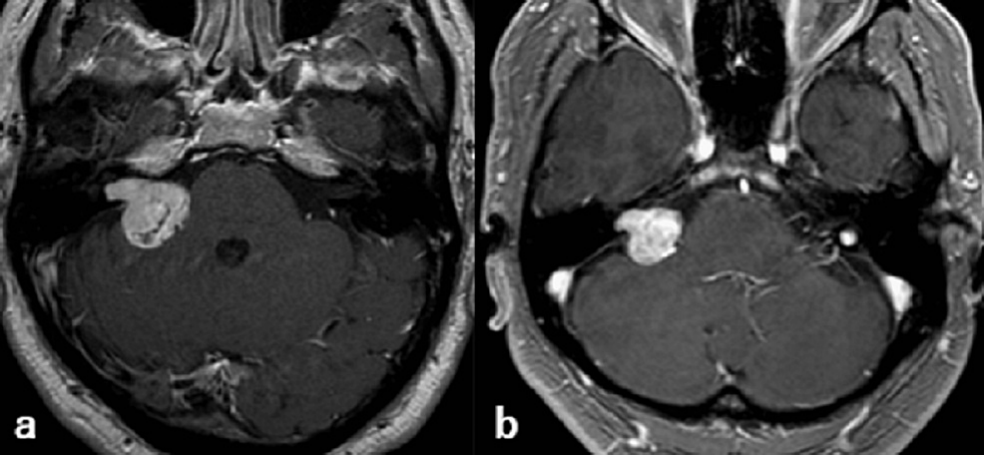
MRI findings - (a) before treatment (b) six years after CyberKnife treatment MRI: magnetic resonance imaging
